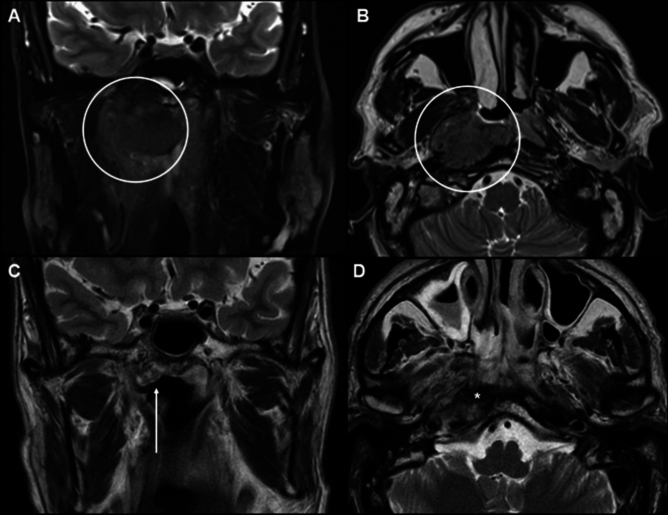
An example of low agreement in T2-weighted imaging evaluation of nasopharyngeal carcinoma (NPC) after radiotherapy. A, B are images before treatment, showing a large NPC with intermediate intensity on the right side of the nasopharynx. C, D are images after treatment, showing resolution of NPC, a hypointense post-radiation scar (asterisk) and a hyperintense soft tissue on the periphery of the scar representing edema (arrow), as confirmed in the subsequent follow-ups. In this case, one expert radiologist and two radiology residents classified the NPC size as reduced, while one expert radiologist and one general radiologist classified the NPC size as disappeared. One radiology resident classified the NPC signal on T2-weighted images as stable, while the other readers classified it as markedly hypointense. The final NI-RADS assigned was 1 for three readers (expert radiologist, general radiologist, and a radiology resident) and 2 for two readers (expert radiologist and a radiology resident)
